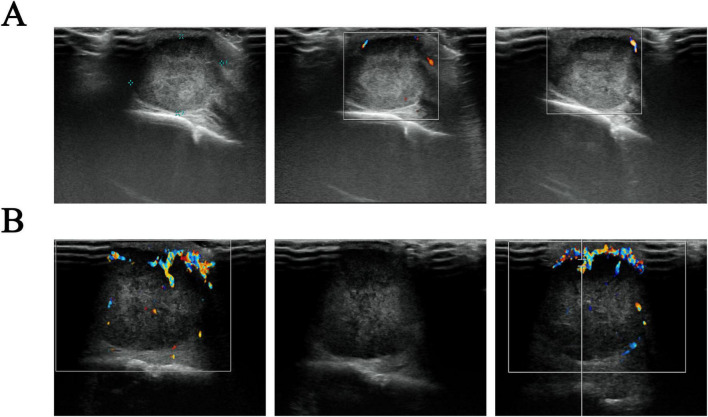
Ultrasound images of a scalp mass. (A) The initial assessment reveals a sub-scalp hypoechoic mass, about 21 × 15 mm in extent. This mass exhibits a defined envelope, regular morphology, and clear boundary, accompanied by uneven internal echogenicity. Color Doppler Flow Imaging (CDFI) indicated no obvious blood flow signal; (B) a re-examination conducted 2 months later suggests that the sub-scalp hypoechoic mass has increased in size to approximately 32 × 24 × 26 mm. The mass now lacks an envelope but still presents with regular morphology and clear boundaries, as well as uneven internal echogenicity. CDFI showed increased blood flow signals both in the periphery and within the mass, characterized by a harmonic frequency pattern indicative of an arterial spectrum.
